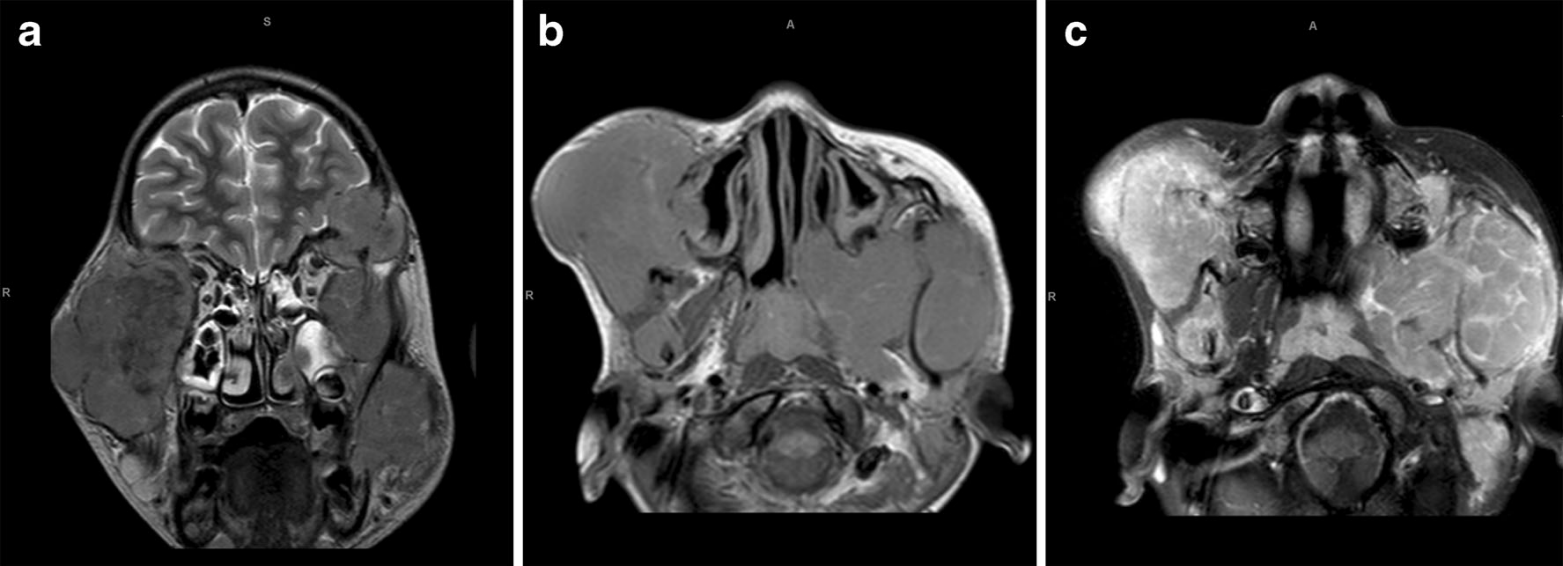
Plasmablastic lymphoma. Coronal T2-W MRI (a) shows multifocal bilateral lobulated facial masses which are predominantly hypointense. On the axial T1-W pre- (b) and post-contrast (c) images there is multi-septated rim enhancement
